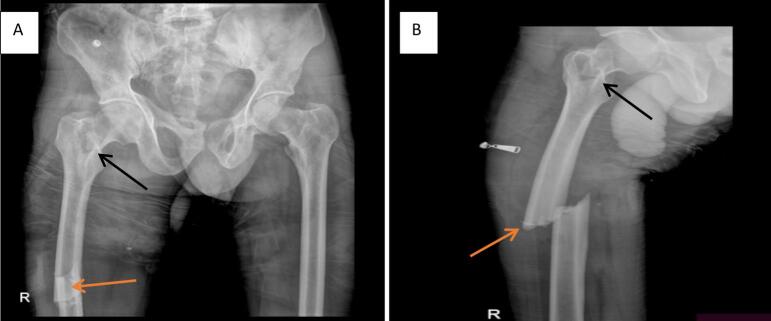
AP (A) and Lateral (B) X-ray views demonstrating a complete transverse femoral shaft fracture with shortening and complete displacement (orange arrows). A lucency consistent with a non-displaced transcervical femoral neck fracture is also visible (black arrows).
